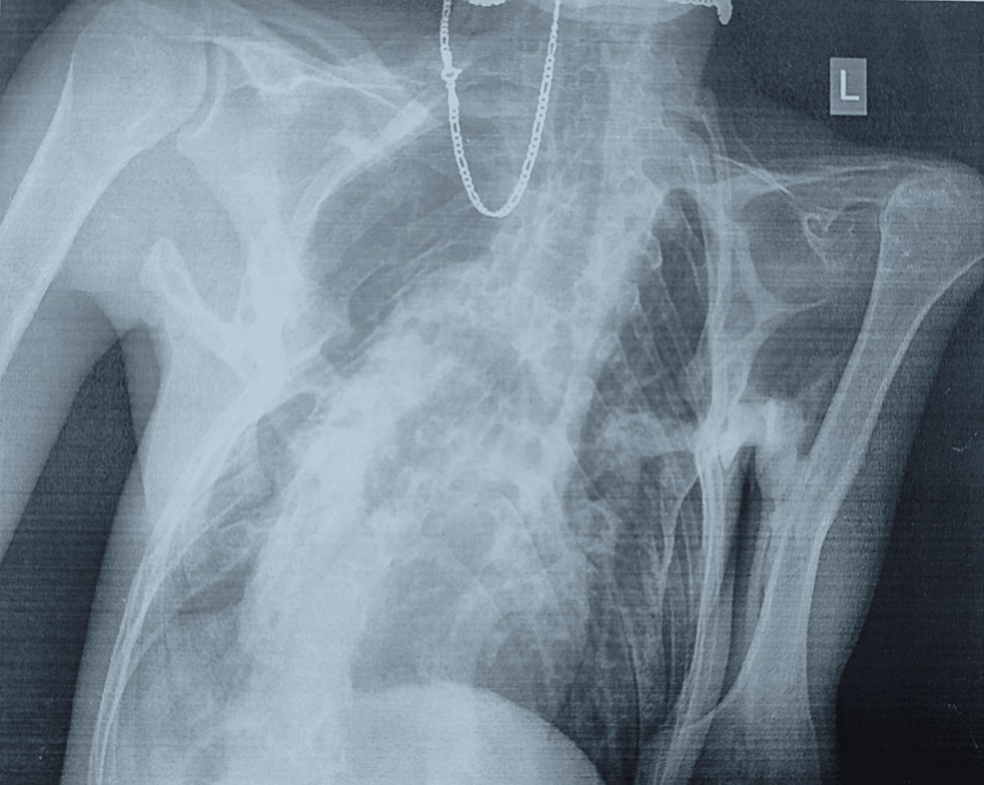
Chest X-ray in the supine position, with analysis significantly limited due to the patient’s forced posture. Fibrotic alteration in the lung parenchyma is observed, with decreased volume bilaterally, which is more pronounced on the right side. Additionally, reduced transparency is noted in the right hemithorax due to a summation effect caused by the patient’s rotation. The depicted segment of the thoracic spine exhibits dextroconvex scoliosis. Coarse shadows of ossifications corresponding to ossified ligaments are seen paravertebrally and in the medial aspect of the thoracic spine. Similar changes are observed bilaterally paravertebrally in the depicted cervical spine section. Ankylosis of the apophyseal joints is visible. In the soft tissues of the right thoracic wall, polymorphic ossifications leading to deformation of the chest wall are observed, with these ossifications merging with the arches of ribs II-VI. Ossifications are also seen in the left thoracic wall’s soft tissues at the axillary projection level, extending toward the left upper arm’s soft tissues and reaching the humeral diaphysis’s cortex.
